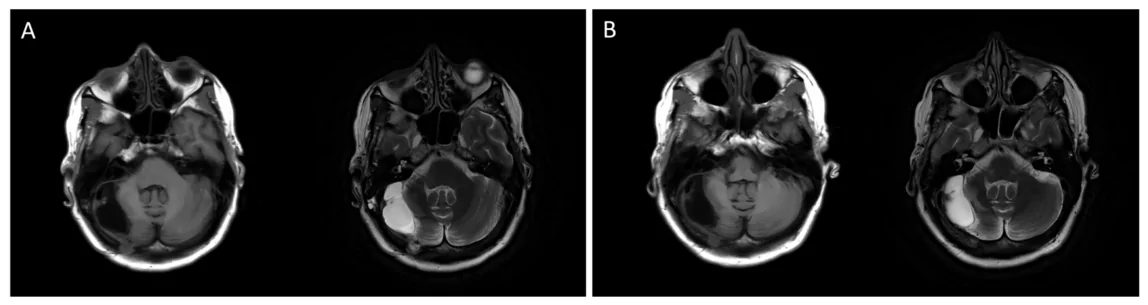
Follow-up brain MRI after local and systemic treatment. (A) November 2025 and (B) February 2026. At each time point, contrast-enhanced axial T1-weighted imaging is shown on the left and axial T2-weighted imaging on the right. Post-treatment findings remained stable without radiographic intracranial progression.
